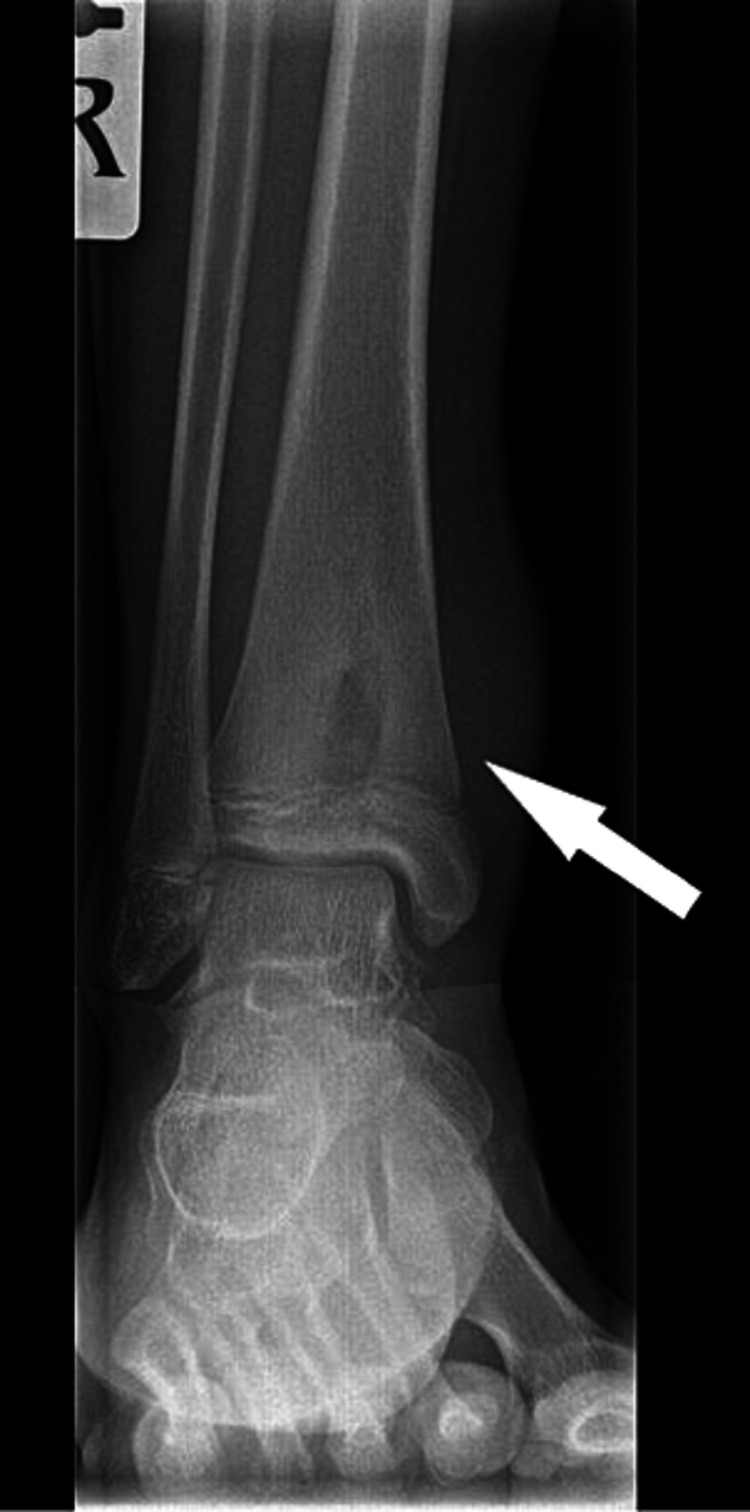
X-ray right distal tibia and fibula AP radiograph showing lesion in the distal tibia involving the physis and metaphysis
